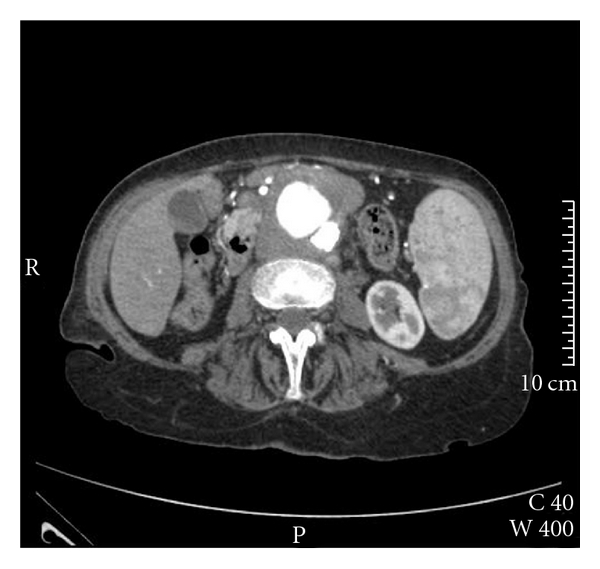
CT scan of the abdominal aorta demonstrating a mycotic aneurysm below the renal arteries.
